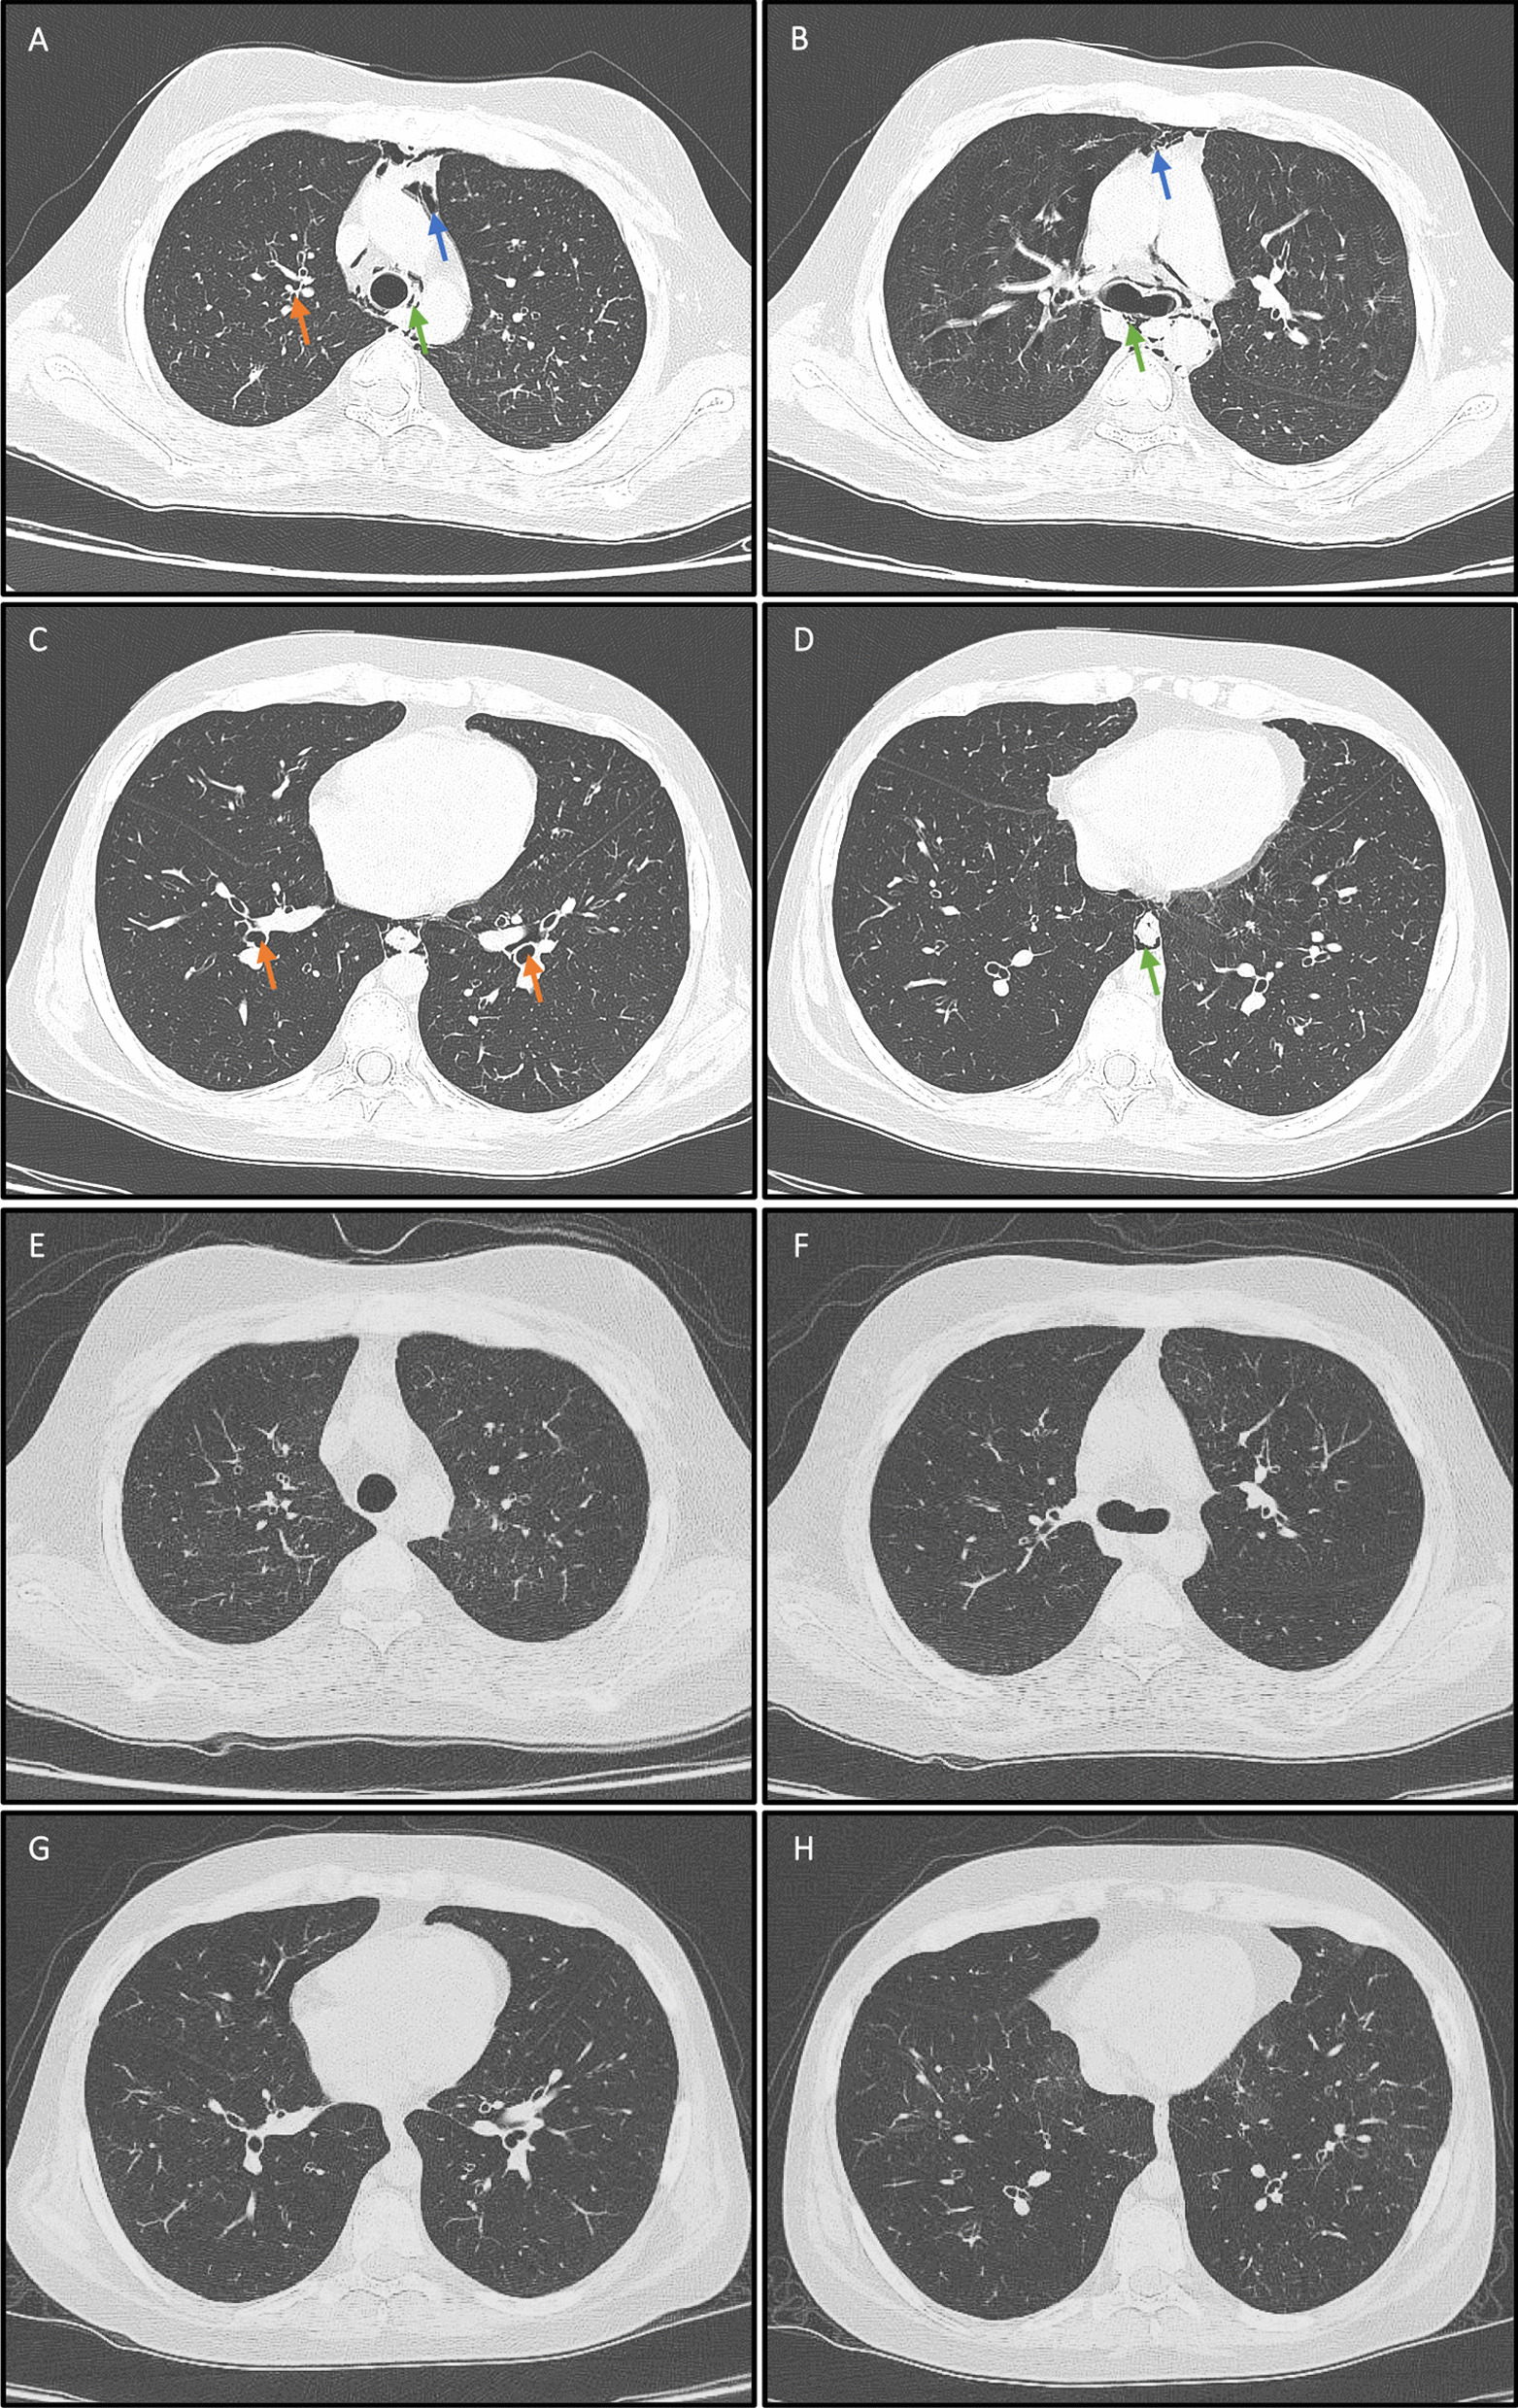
Chest CT scan images of the first patient before (panels A–D) and 12 months after (panels E–H) treatment with AT-MSCs. Resolution of bilateral lung hyperaeration, pneumomediastinum (*green arrows*), pneumopericardium (*blue arrows*), and to a lesser extent, bronchiectasis (*orange arrows*) is evident
